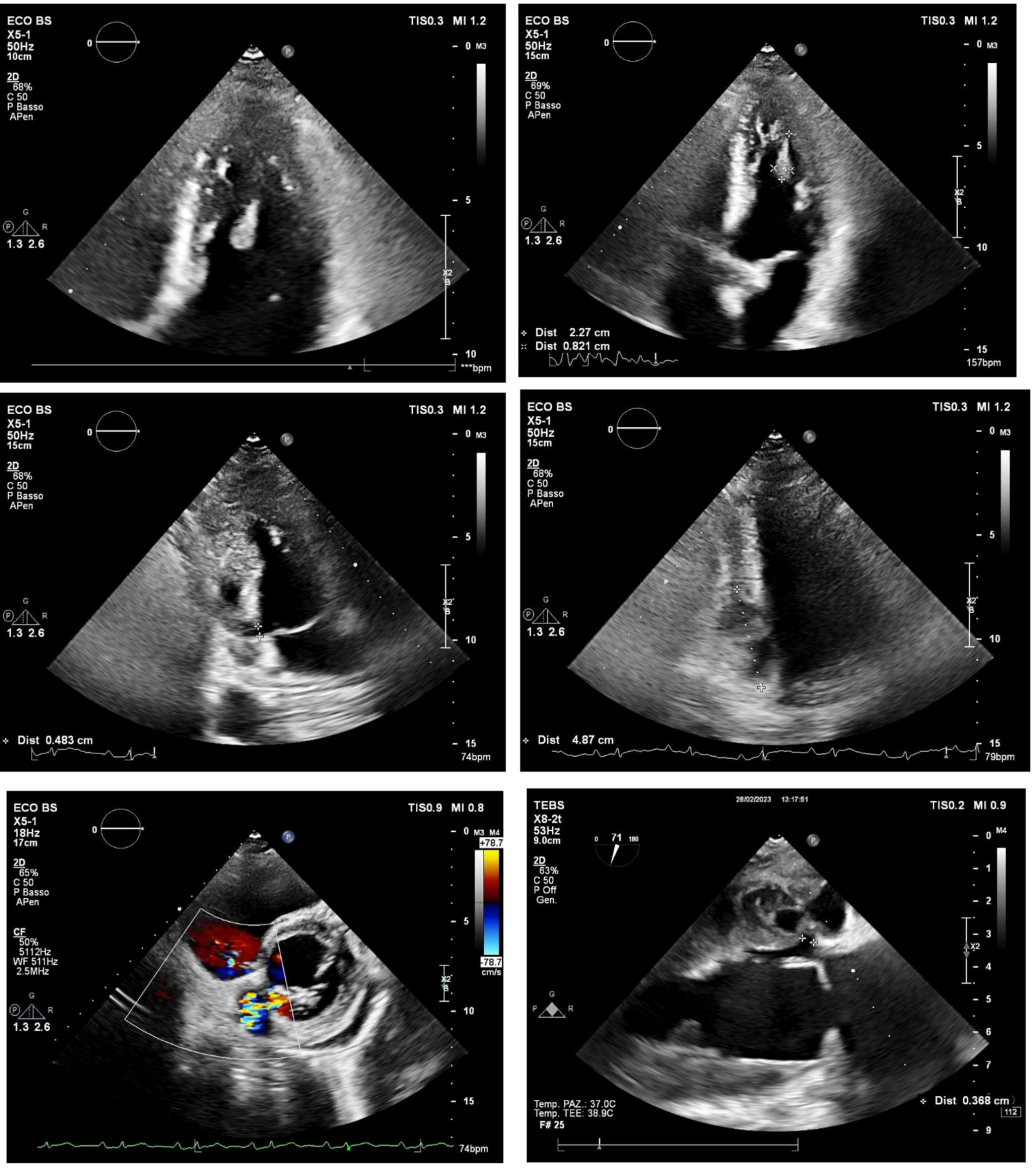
Floating pedunculate formation in the left ventricle and intramiocardial abscess seen at TTE in different echocardiographic projections
